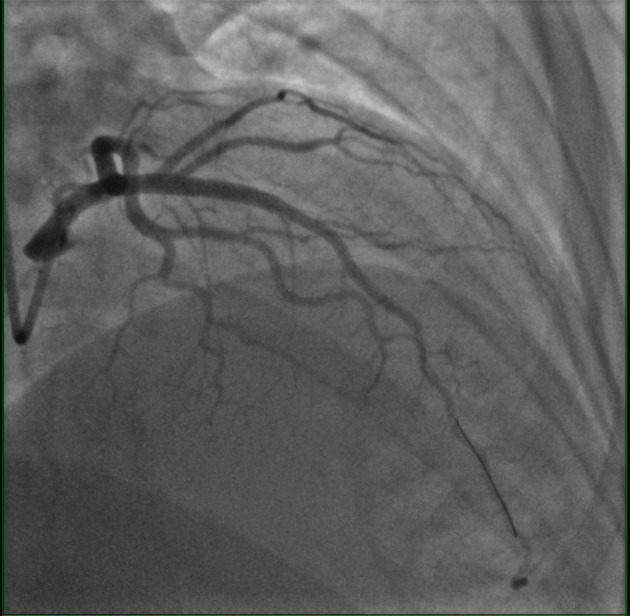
Post-intervention coronary angiography following intravascular ultrasound (IVUS)-guided percutaneous coronary intervention (PCI) with placement of a single drug-eluting stent in the mid left anterior descending coronary artery (LAD). The stent demonstrates complete expansion without geographic miss, residual stenosis, or edge dissection. TIMI-3 anterograde flow is restored throughout the mid and distal LAD and its diagonal branches, with no evidence of distal embolization or no-reflow phenomenon. The post-dilation result, achieved with a 4.0 × 15 mm non-compliant balloon at 14 atmospheres, confirms adequate luminal gain and stent apposition.
